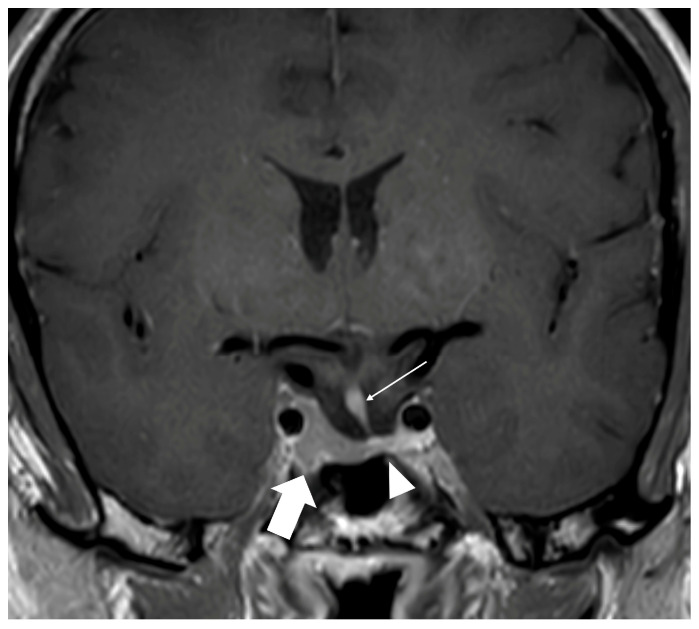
Coronal MRI section showing pituitary left-sided apoplexy (arrowhead) with right-sided enlargement (bold arrow), partial surrounding of the carotid artery (Knosp grade II) and deviation of the pituitary stalk (arrow).
